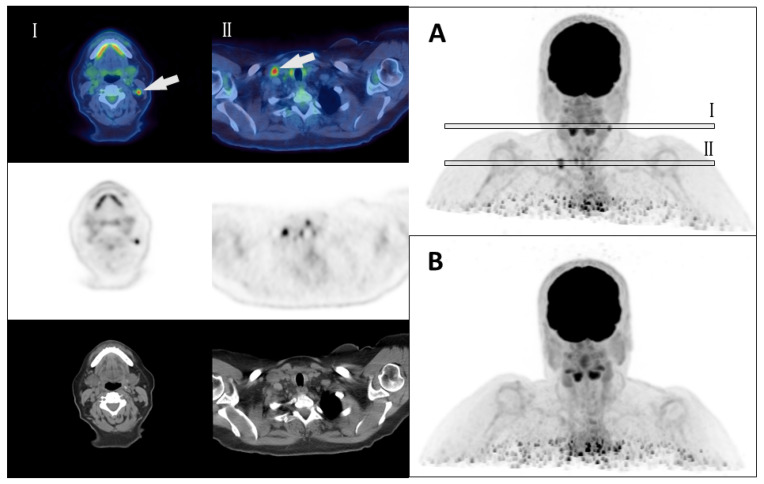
Example of pre- and post-therapeutic [^18^F]FDG-PET/CT findings. Pretherapeutic [^18^F]FDG-PET/CT imaging (A) shows multiple focal FDG lesions (n = 5) classified as lymph node metastases in a patient with high-risk papillary thyroid cancer (T3, N1, R1) in the 3D-PET-reconstruction. Two of these metastases are marked with a white arrow in cross-sections (I) and (II). Decision for PET/CT was made due to a mismatch of a high hTg Level of 72.5 ng/mL (endogen TSH stimulation) and only little iodine-avid tissue in the first radioiodine treatment. Following PET/CT, a cervical lymph node dissection was performed confirming multiple lymph node metastases (n = 6). A second [^18^F]FDG-PET/CT imaging after surgery demonstrated the absence of any FDG-avid lesions (B) and hTg dropped below the detection limit until the end of the follow-up period (73 month from resection).
